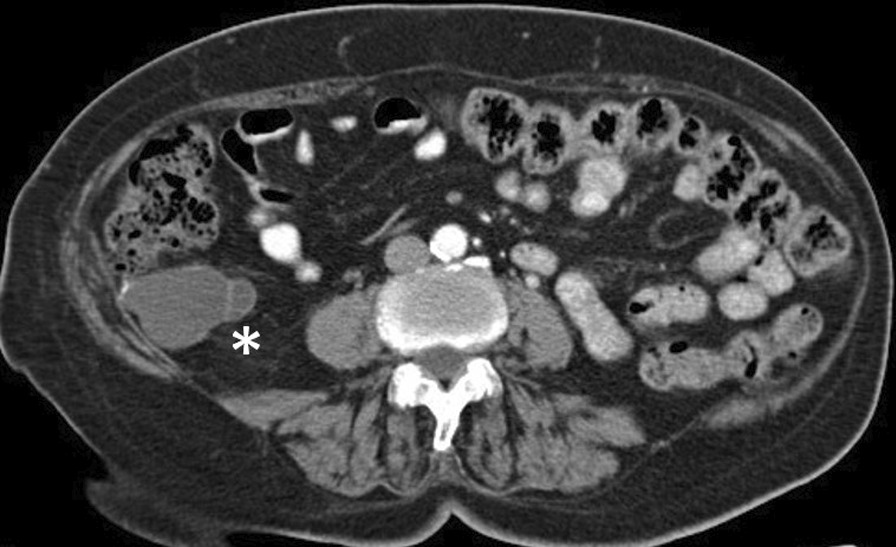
CT scan showing low-grade appendiceal mucinous neoplasms with liquid density adjacent to the caecum and retrocecum (*)
